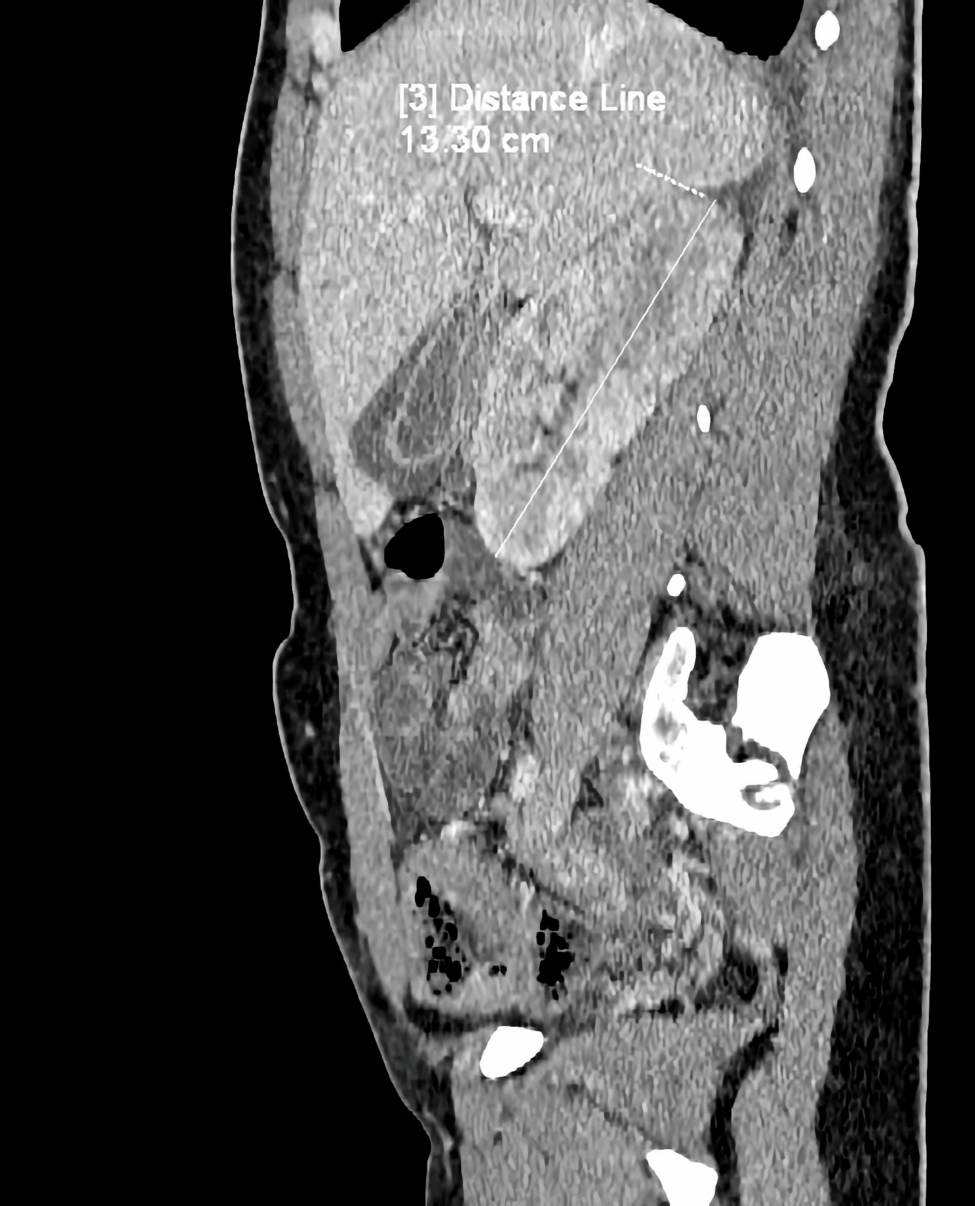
CT scan (sagittal) showing enlarged right kidney and pericholecystic inflammatory changes CT: computed tomography
